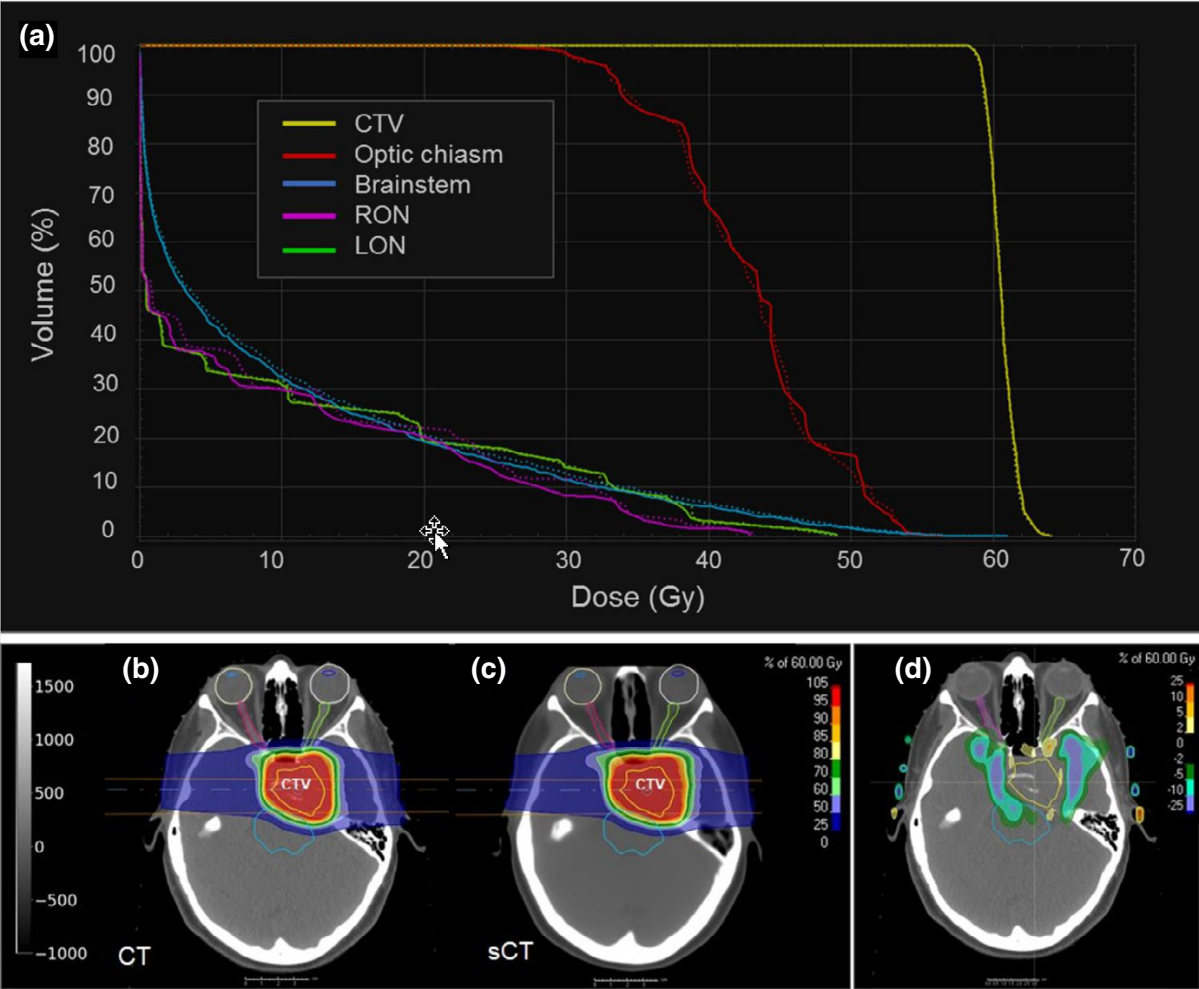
Dose volume histogram (a) for test patient #5 representing the pencil beam doses computed in computed tomography (CT) (solid line) and synthetic CT (dotted line) for the clinical target volume and the following organs at risks: right optic nerve, left optic nerve, brainstem, and optic chiasm. The corresponding dose distributions (at one slice in the center of the target) are presented in (b) for CT, (c) for sCT, and (d) the dose difference.
